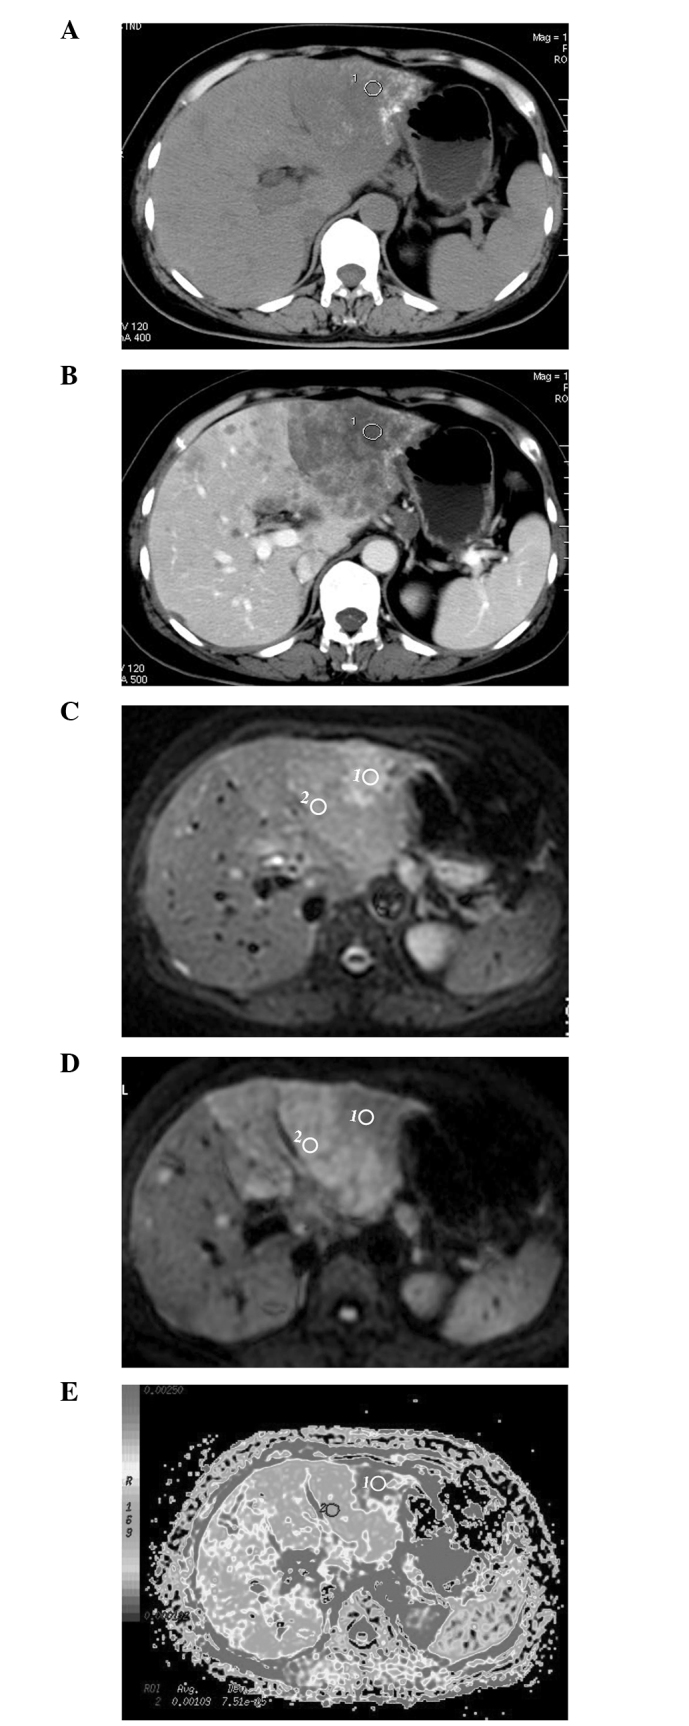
Follow-up CT images eight weeks after lipiodol-based chemoembolization for a 52-year-old female with hepatocellular carcinoma in the left hepatic lobe. CT scan revealed faint accumulation of iodized oil (type III) in the (A) lesion and (B) residual viable tumor with contrast enhancement. Diffusion-weighted magnetic resonance images obtained using b values of (C) 0 and (D) 500 sec/mm^2^. (E) ADC map calculated from diffusion-weighted images. Necrotic components of the tumor (1O) showed greater signal attenuation and higher ADC values compared with the cellular enhancing viable components of the tumor (2O), which restricted diffusion. CT, computed tomography; ADC, apparent diffusion coefficient.
